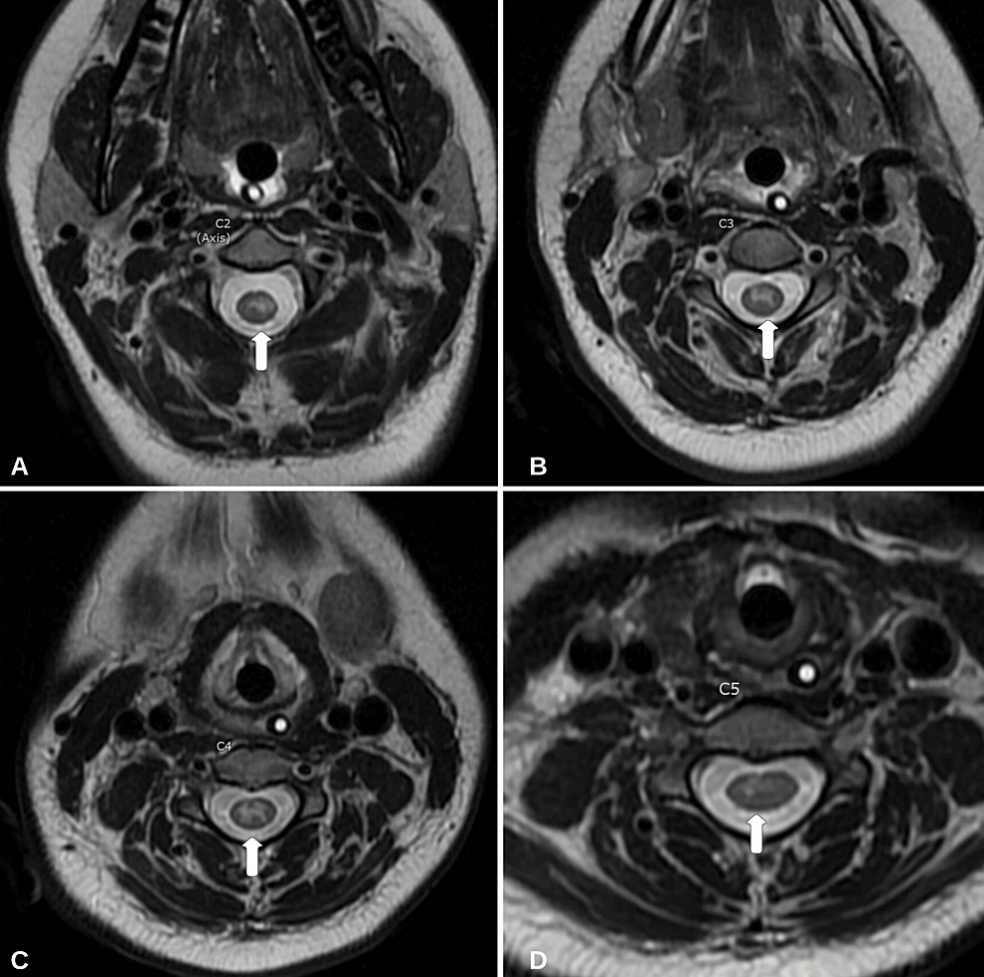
MRI (axial view) of the cervical spine (A-D) T2 FLAIR (axial view) of spinal segments C2-C5 reveals primarily gray matter hyperintensities (arrows). MRI: magnetic resonance imaging, FLAIR: fluid-attenuated inversion recovery
